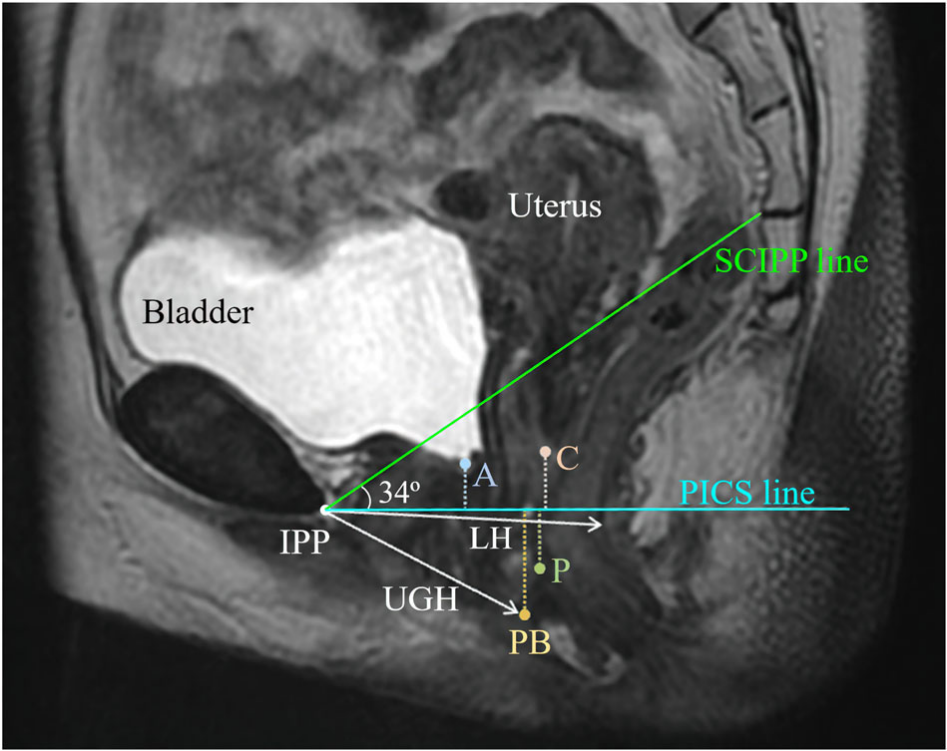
Measurements made in the midsagittal plane during magnetic resonance imaging (MRI). A, lowest point of the anterior vaginal wall; C, lowest point of the uterine cervix or vaginal stump, P, lowest point of the posterior vaginal wall, PB, front edge of the perineal body, SCIPP line, sacrococcygeal inferior pubic point line; PICS line, pelvic inclination correction line; LH, levator hiatus; UGH, urogenital hiatus
